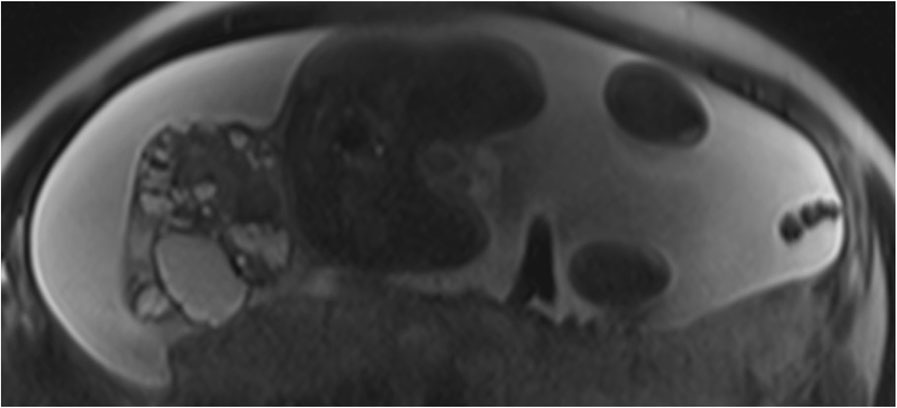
Same MRI, coronal cut of the mixed mass
